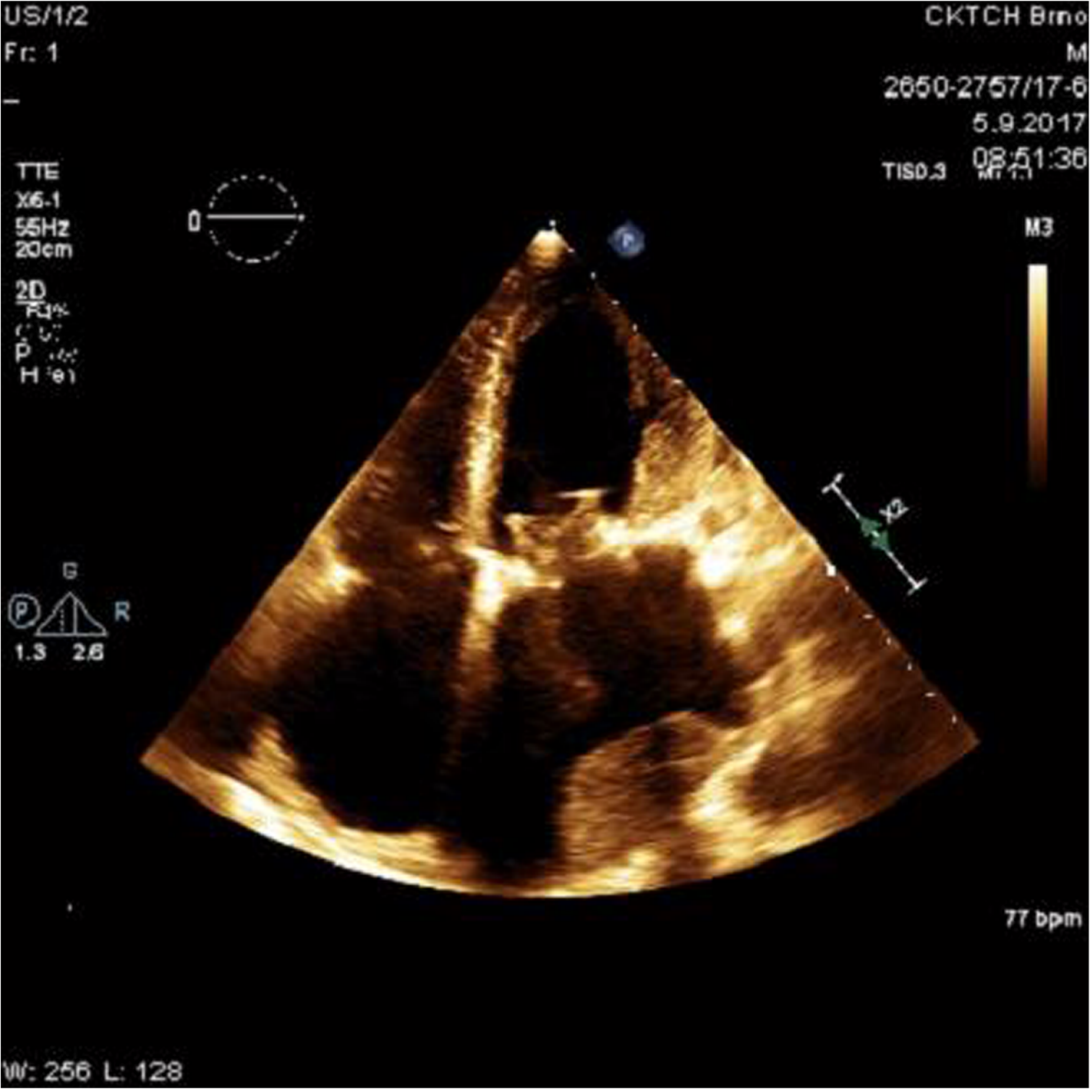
Echocardiogram 3 months post-surgery An apical four-chamber view showing an unknown left atrial mass attached to the posterior wall of the left atrium
